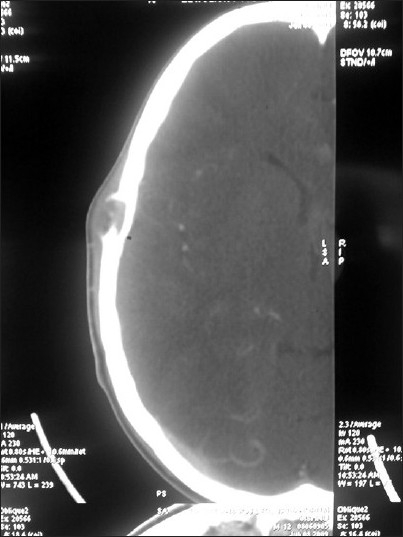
CT head, axial section, shows an osteolytic lesion in the right high temporal region, with interruption of the outer table and an intact inner table of the skull
